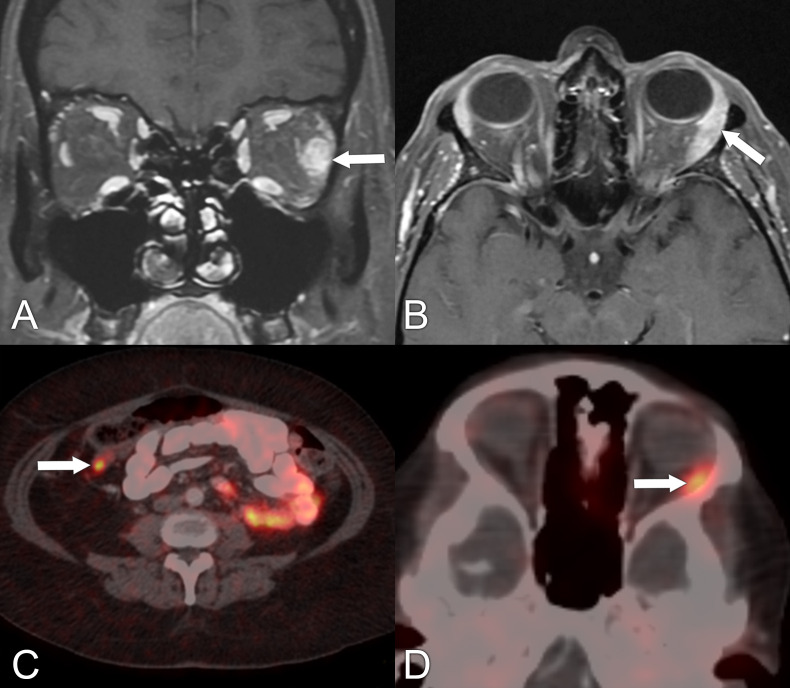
Magnetic resonance imaging and ^68^Ga-DOTATATE PET/CT in a 68-year-old woman with carcinoid tumor of the ileum with metastasis to the left orbit. (A) Coronal T1 postcontrast MRI with fat saturation shows a mass in the belly of the left lateral rectus muscle (arrow). (B) Axial T1 postcontrast MRI with fat saturation shows the lesion in the left lateral rectus muscle belly (arrow). (C)
